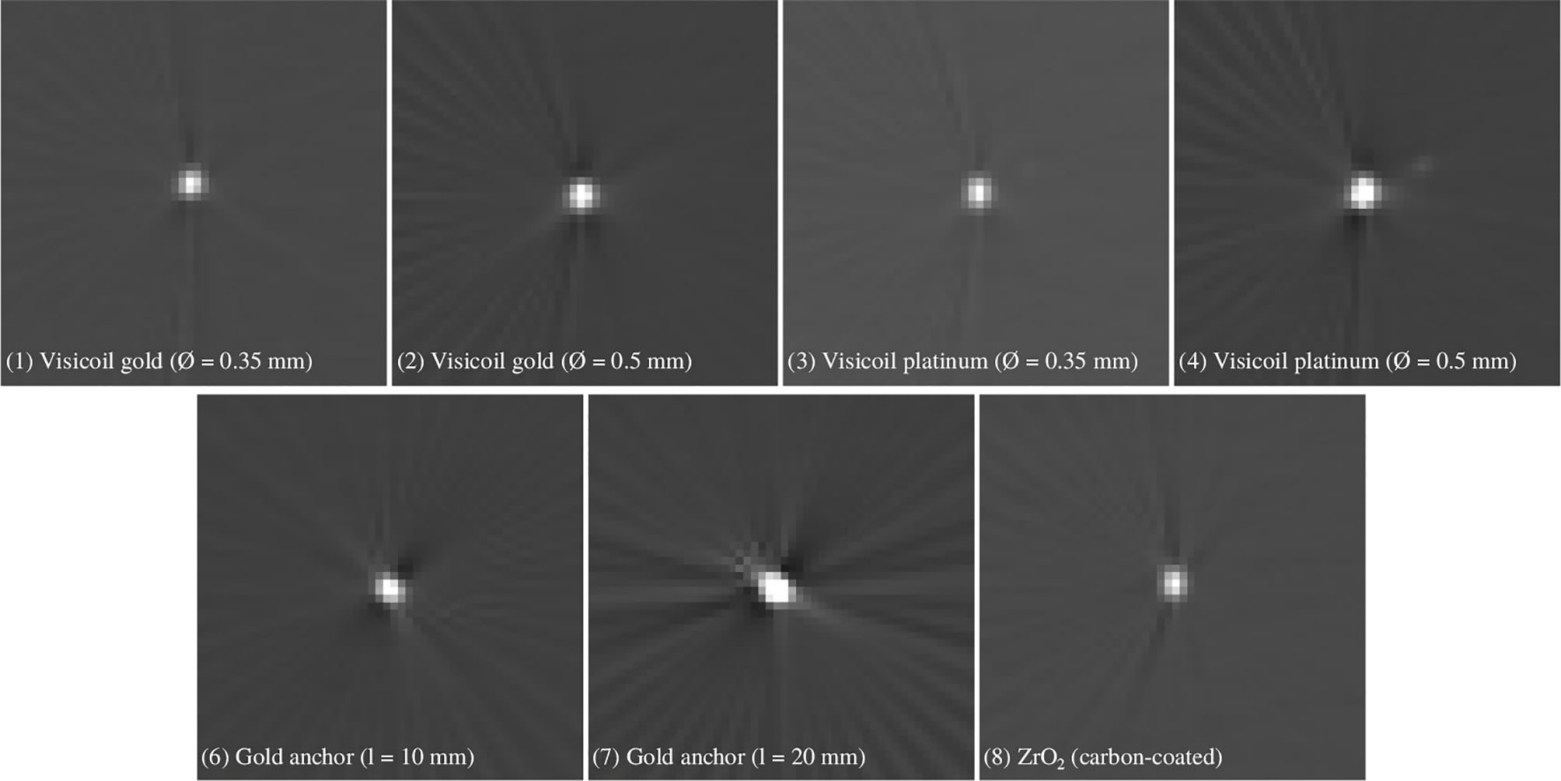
In-room mobile CT images of 7 different fiducial markers listed in Table 1.
